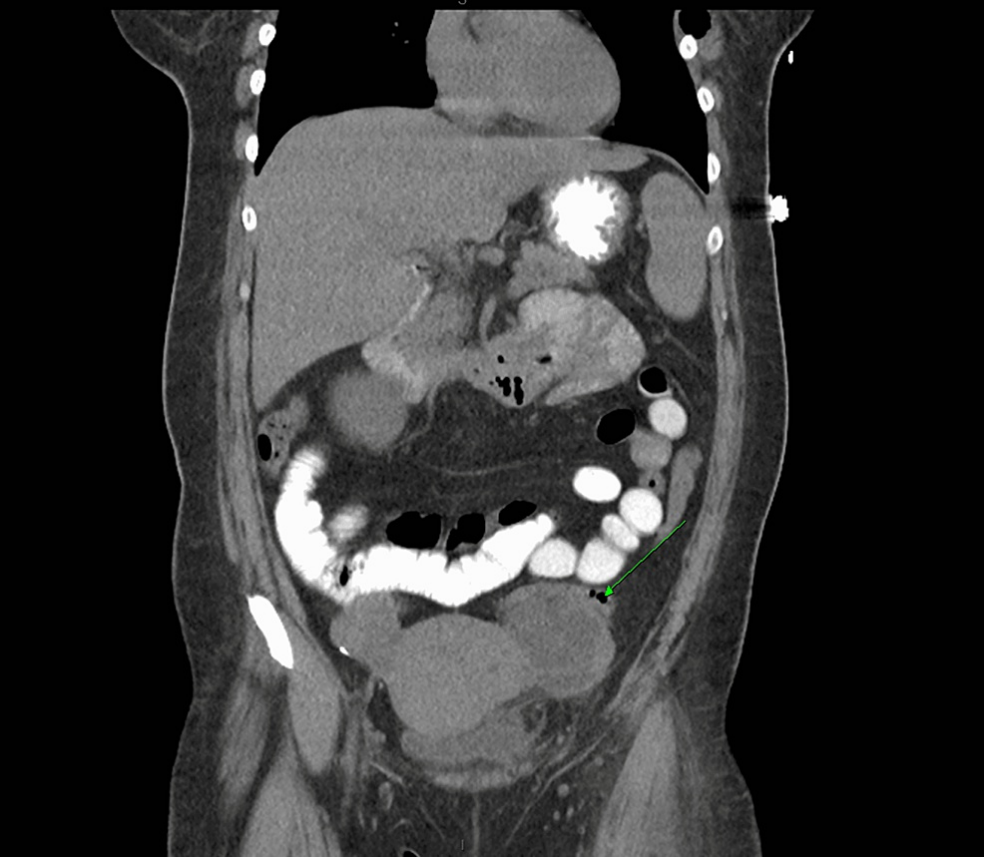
Computed tomography without contrast of the abdomen in coronal view. The green arrow indicates left ovarian mass.
